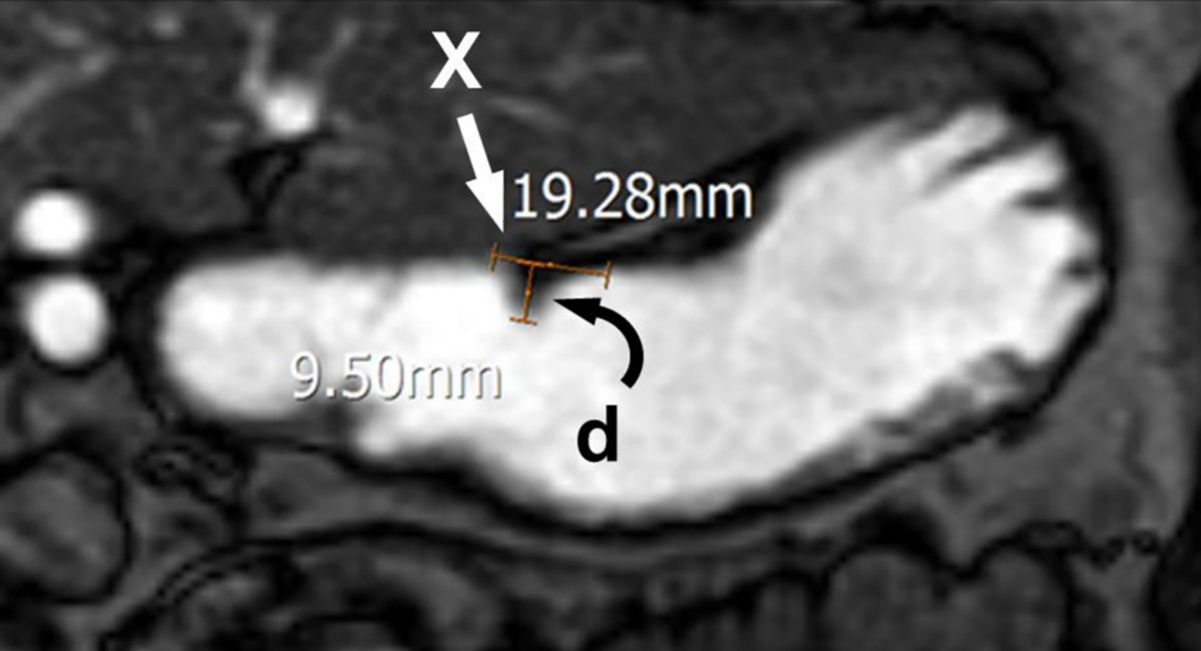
Measurements for calculating gastric motility index of a 68-year-old man with Parkinson’s disease. Wavelength (X, straight arrow) and amplitude (d, curved arrow) of gastric peristaltic waves were measured in the 3D balanced-turbo field echo MR image. Number of waves (not shown in this image) was also obtained from the same sequence. Gastric motility index was calculated based on these measurements.
